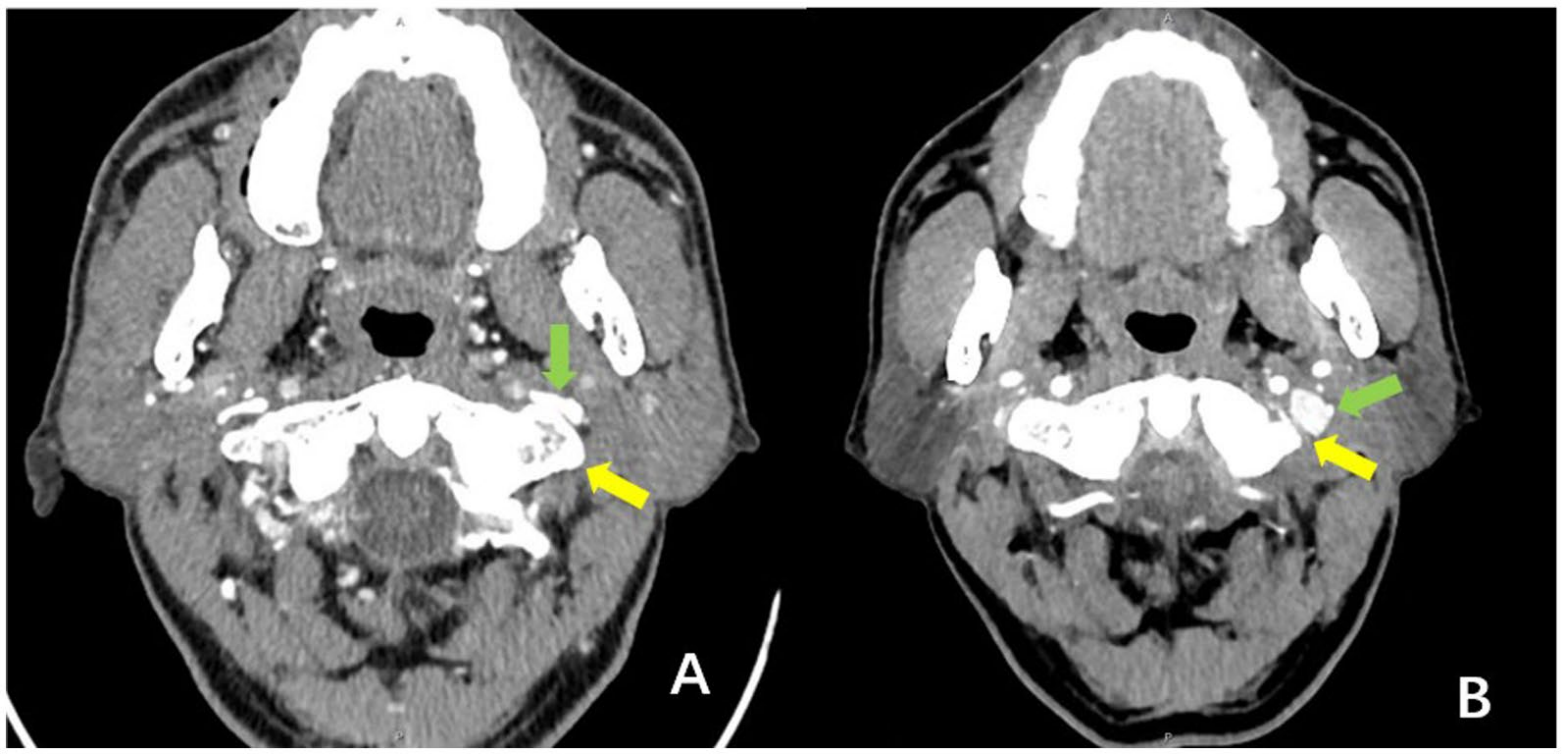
Comparative morphology of the internal jugular vein in a representative patient before surgery and 3 months postoperatively (axial CT venography views). (A) Preoperative image: Demonstrates protrusion of the atlas transverse process (yellow arrow), causing direct compression and significant luminal stenosis of the ipsilateral internal jugular vein (green arrow). (B) Postoperative (3-month) image: Shows resection of the compressive portion of the atlas transverse process (yellow arrow). The previously compressed segment of the internal jugular vein (green arrow) now exhibits substantial luminal expansion and restored morphology. Interpretation: This comparison provides direct visual evidence of successful surgical relief of the bony compression and subsequent anatomical restoration of the jugular vein.
